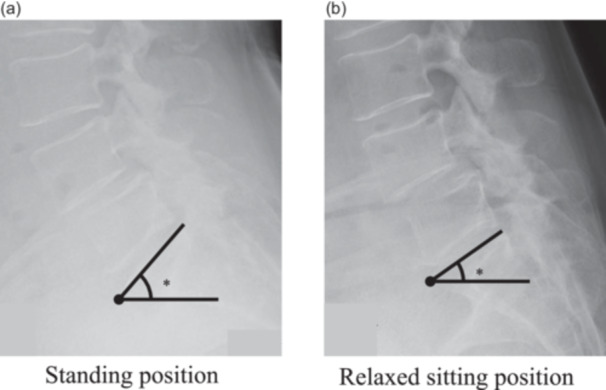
Radiographic evaluation of sacral slope in two postures, obtained preoperatively and at final follow‐up. (a) Evaluation in a natural standing position. (b) Evaluation in a relaxed sitting position. Sacral slope (*) is measured as the angle between the superior endplate of S1 and a horizontal reference line (solid lines).
